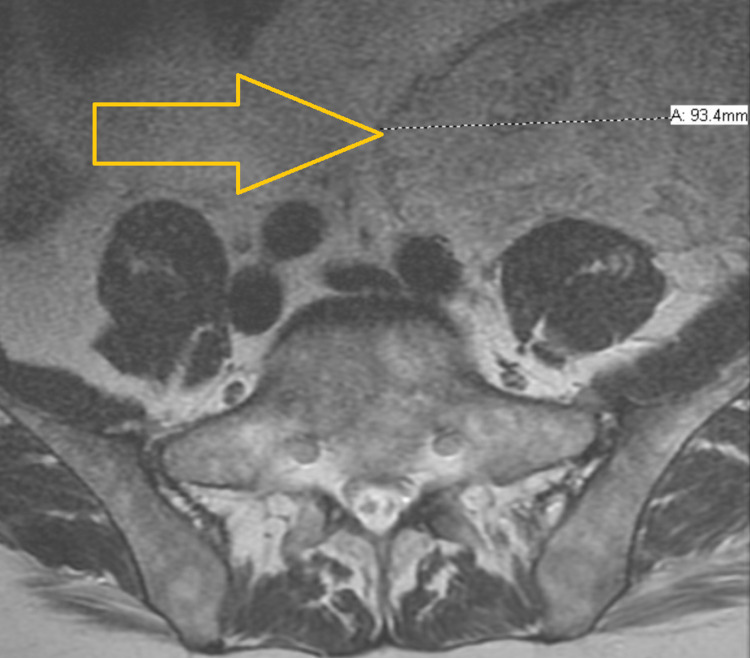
MRI of the spine showing a very large, heterogeneously enhancing, mixed-signal, mass-like lesion in the left hemipelvis. MRI: magnetic resonance imaging
